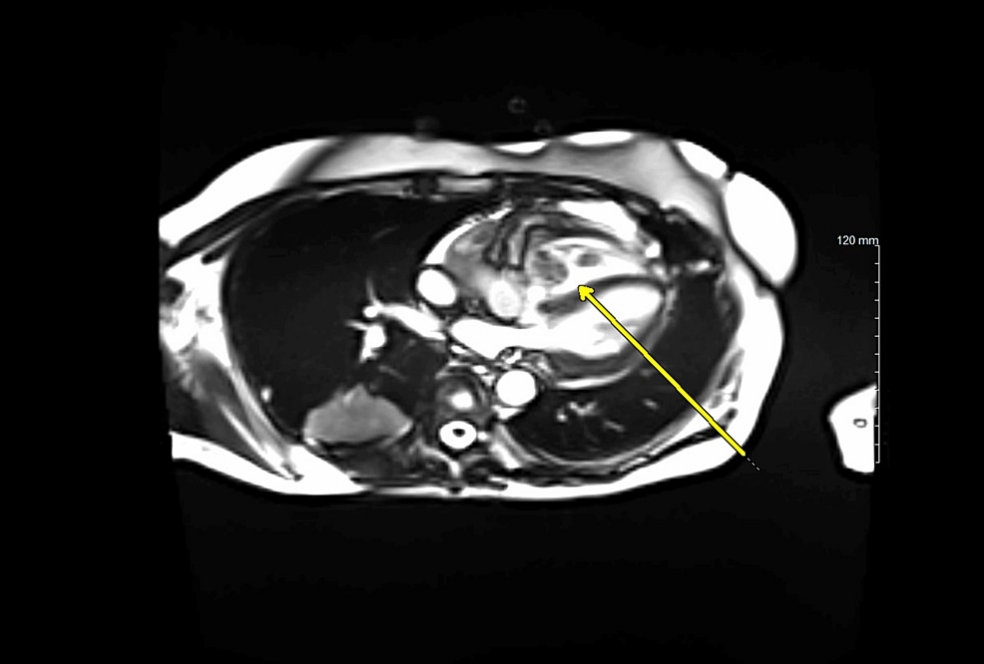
Multilobular right ventricular mass on cardiac MRI, later identified as diffuse large B-cell lymphoma
